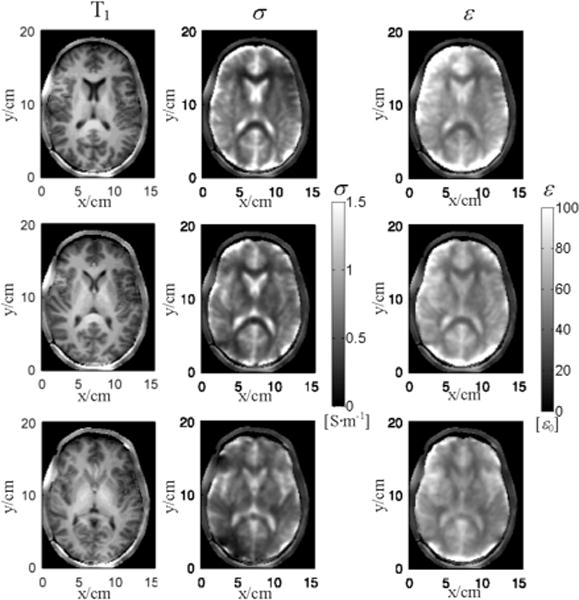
Reconstructed *in vivo* EPs using the gradient-based EPT algorithm in three slices of a healthy human subject’s brain. Left: reference T1-weighted images; middle: reconstructed conductivity images; right: reconstructed permittivity images. Reproduced with permission from Liu et al., 2015; 74: 634–646.
